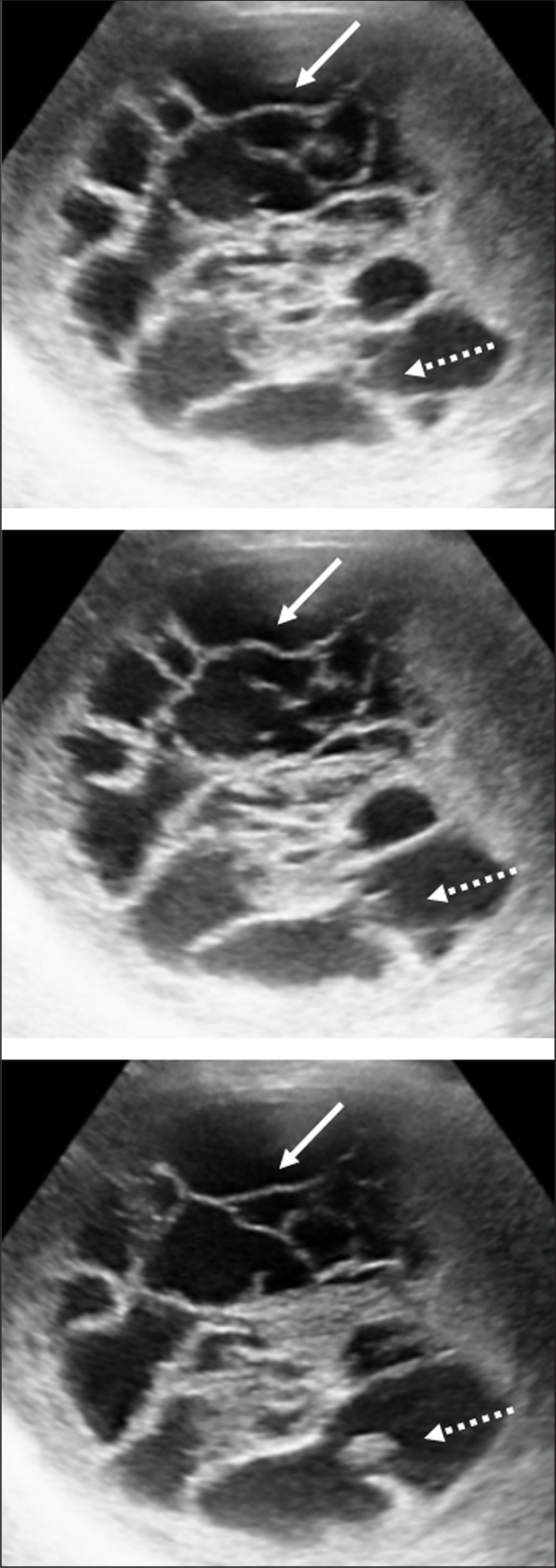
A CT scan without and after contrast enhancement showed a voluminous cystic lesion of 13 × 11 × 11 cm, with regular contours occupying the right hepatic dome. However, the multiple hyperechogenic mobile septa detected on ultrasonography (Figure 2a) were not visualized, even with enhanced CT (Figure 2b). As in the ultrasound examination, there was a non-enhancing gravity-dependent hyperdensity (33 Hounsfield unit), corresponding to a blood clot (Figure 2b). These findings are typical of a hemorrhagic hepatic cyst (HHC).
